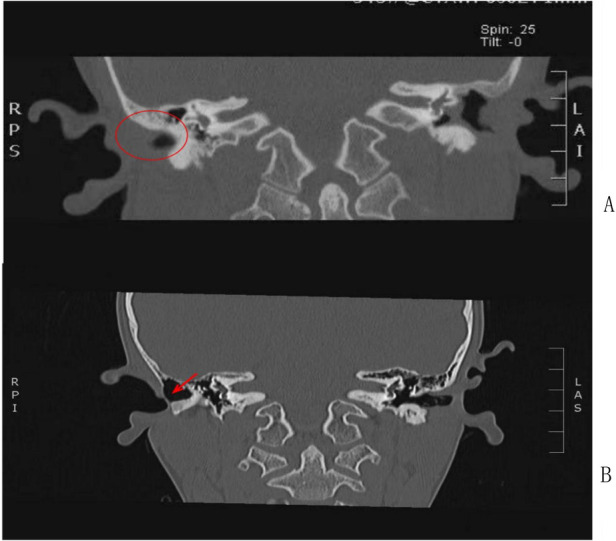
(A) temporal bone CT showing complete obliteration of the right EAC lumen by dense fibrous scar tissue, with no visible tympanic membrane. (B) The EACH developed 6 months after modified radical mastoidectomy (MRM) for chronic suppurative otitis media and mastoiditis. High-resolution temporal bone CT (axial view) showing linear fibrous obstruction of the right bony EAC with a residual lumen diameter of 1.5 mm, no bony hyperplasia or mastoid air cell involvement.
